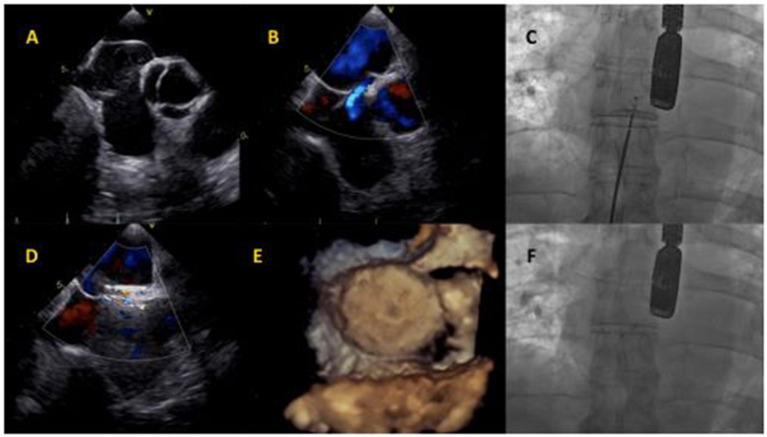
Cocoon PFO Occluder implantation. (A) Baseline transesophageal echocardiography showing atrial septum aneurysm and Chiari network. (B) Baseline interatrial shunt. (C) Cocoon PFO Occluder positioning at fluoroscopy. (D) Intraprocedural transesophageal echocardiography, showing minimal intra-device shunt. (E) Device assessment with 3D-echocardiography imaging. (F) Final device release at fluoroscopy.
